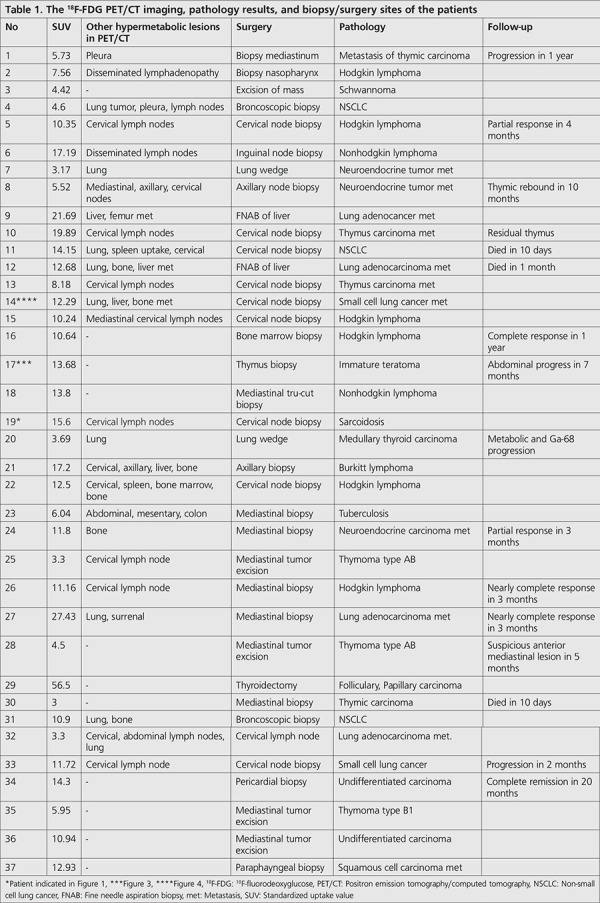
The ^18^F-FDG PET/CT imaging, pathology results, and biopsy/surgery sites of the patients
